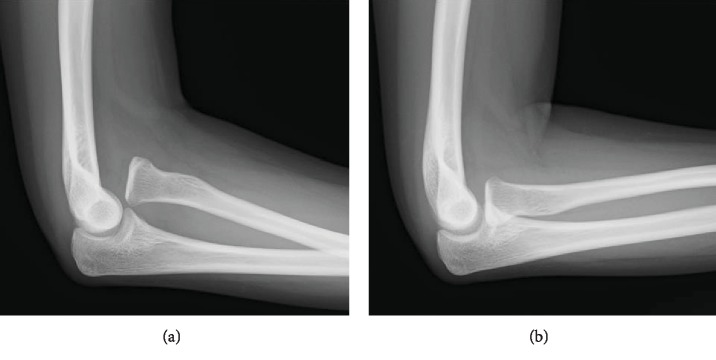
Preoperative radiographic images. The lateral radiographs show anterior dislocation of the radial head (RH) with the forearm in supination (a) and complete reduction of the RH in pronation (b).
